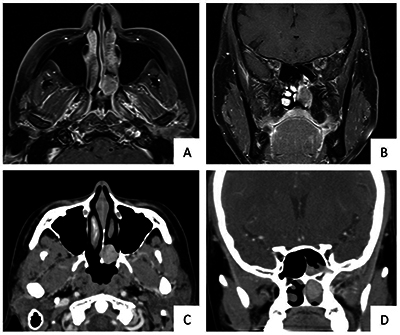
Atypical location of olfactory neuroblastoma in a 36-year-old woman presenting with nasal obstruction for 6 months. Contrast-enhanced T1-weighted imaging with fat suppression (A-B) and contrast-enhanced computed tomography (C-D) showed an enhanced mass with an epicenter at the middle part of left nasal cavity.
